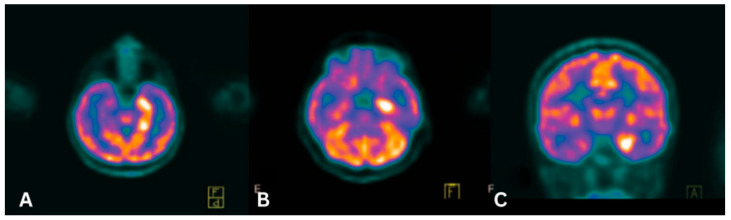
(A,B) Axial and (C) coronal: FDG PET/CT image shows significant asymmetric hypermetabolism of the bilateral mesial temporal lobes, consistent with limbic encephalitis. Clinical takeaway: PET/CT can reveal metabolic changes supporting PLE even when anatomic imaging is equivocal. Source [19].
